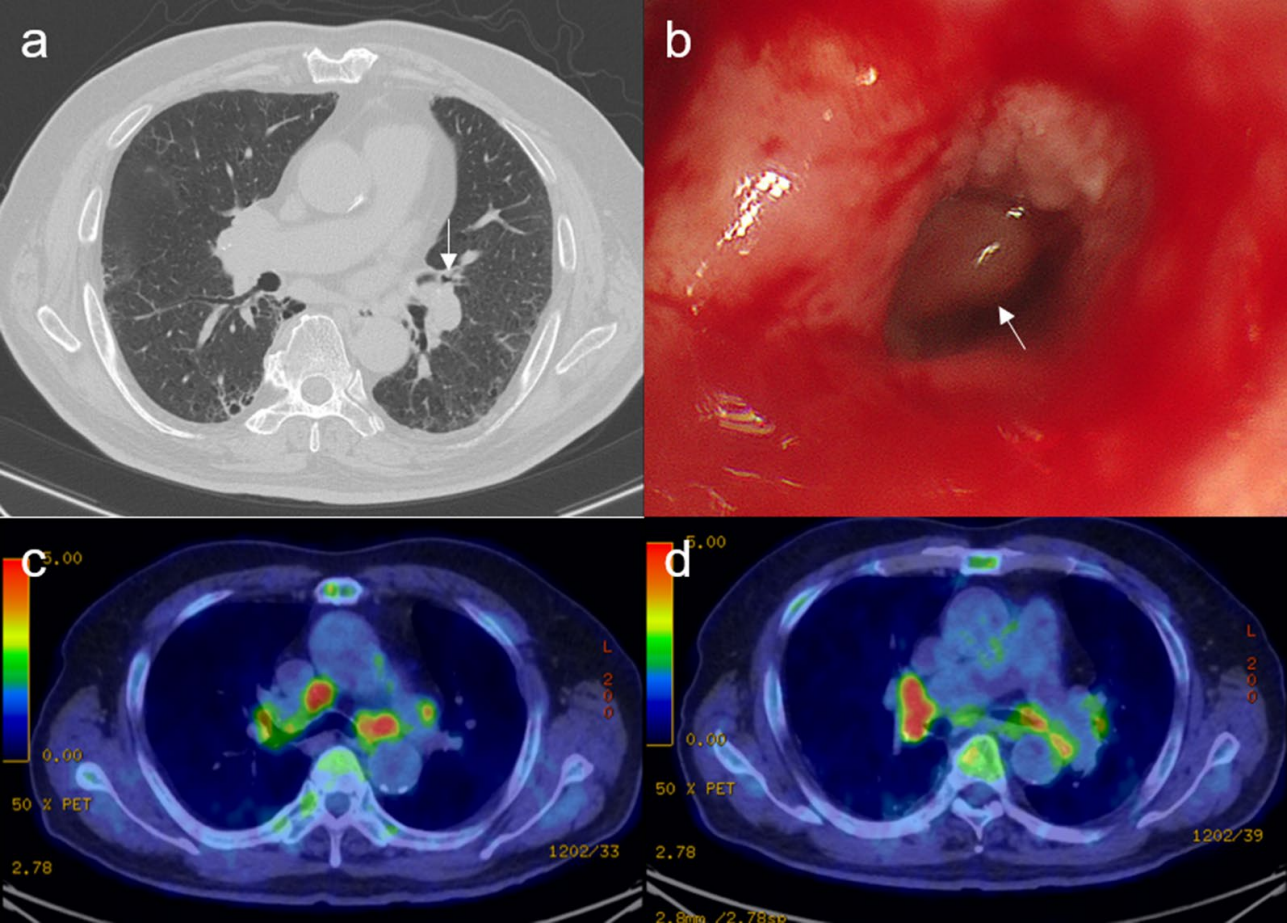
Preoperative findings. a Computed tomography shows a polypoid nodular shadow in the left superior lingual bronchus (white arrow) without lymphadenopathy. b Bronchoscopy shows a polypoid nodule in the left superior lingual bronchus (white arrow). c, d Accumulation of 2-deoxy-2-(18F)-fluorodeoxyglucose up to the maximal standardized uptake value of 6.0 is observed in the bilateral hilar and mediastinal lymph nodes
